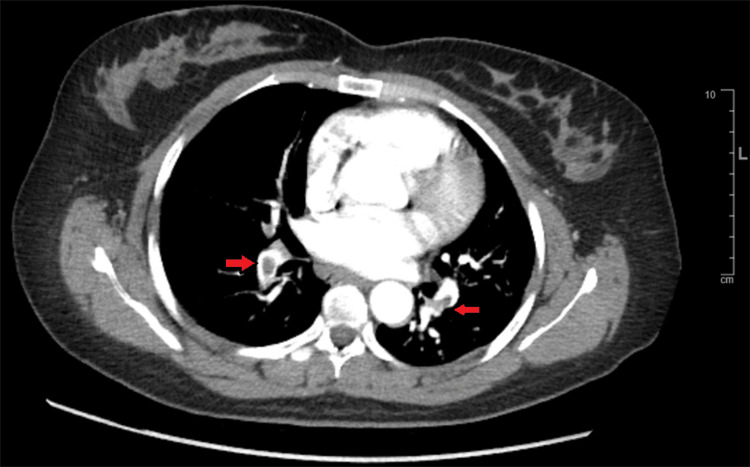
CT scan of the chest showing bilateral pulmonary embolism (red arrows).
